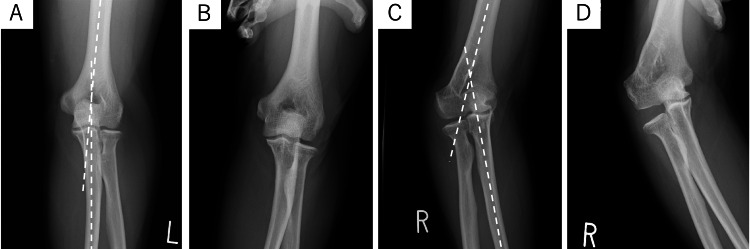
Preoperative radiographs of case two (A) Left AP radiograph. The carrying angle (CA) was 5 degrees in the left upper extremity. (B) The left elbow showed no instability on varus stress testing. (C) Right AP radiographs showed a cubitus varus deformity of 25 degrees. (D) Right AP radiograph under varus stress. The right elbow showed 15 degrees of varus instability.
